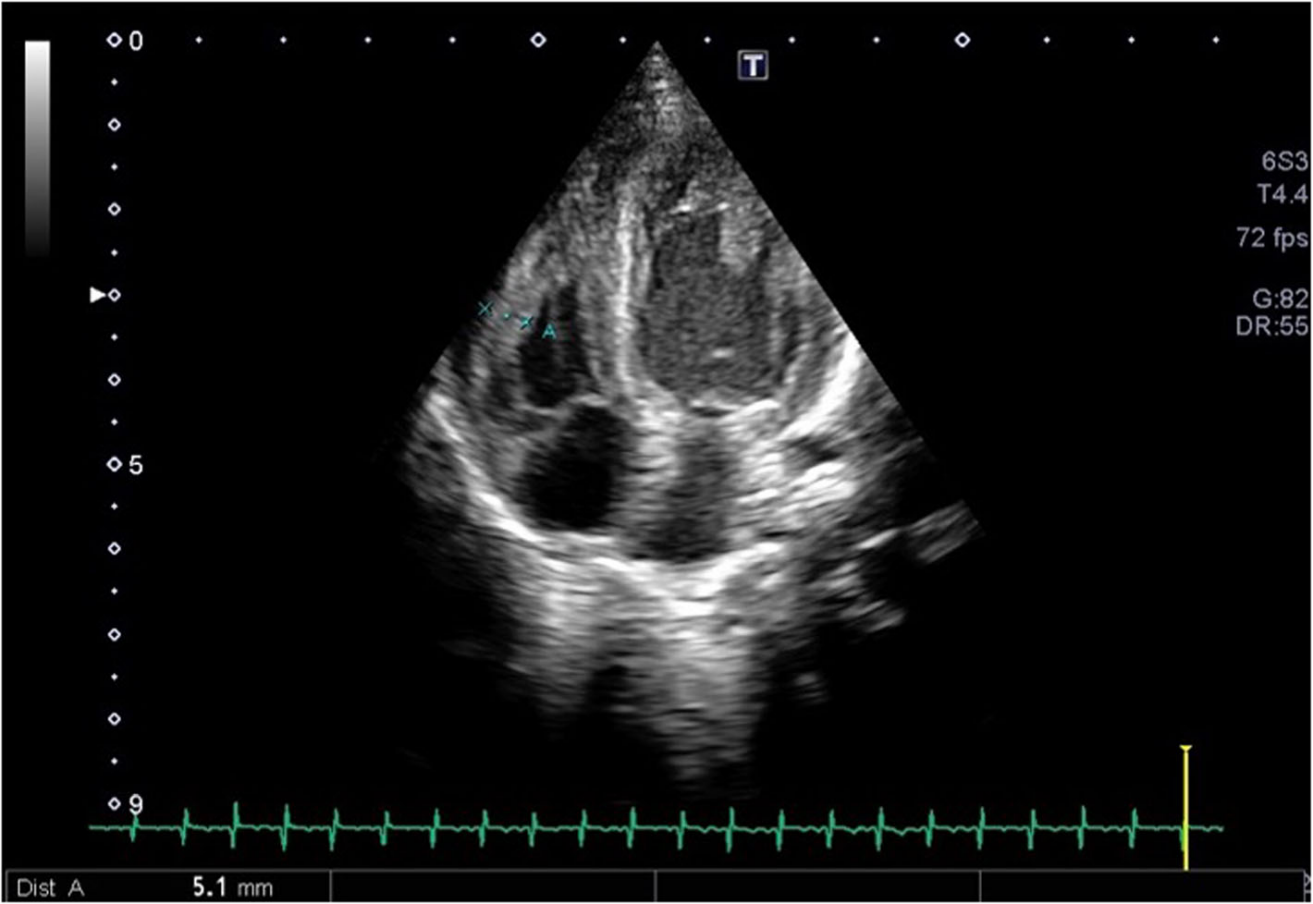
Echocardiogram performed (3 months of age) showed an interventricular septal hypertrophy (SIVd 11.2 mm; z-score 5.1) and a significant reduction in left ventricular cavity (EDV 5 ml) with ecocardiographic signs of LV outflow tract obstruction (maximum intraventricular pressure gradient 7.9 mmHg)
